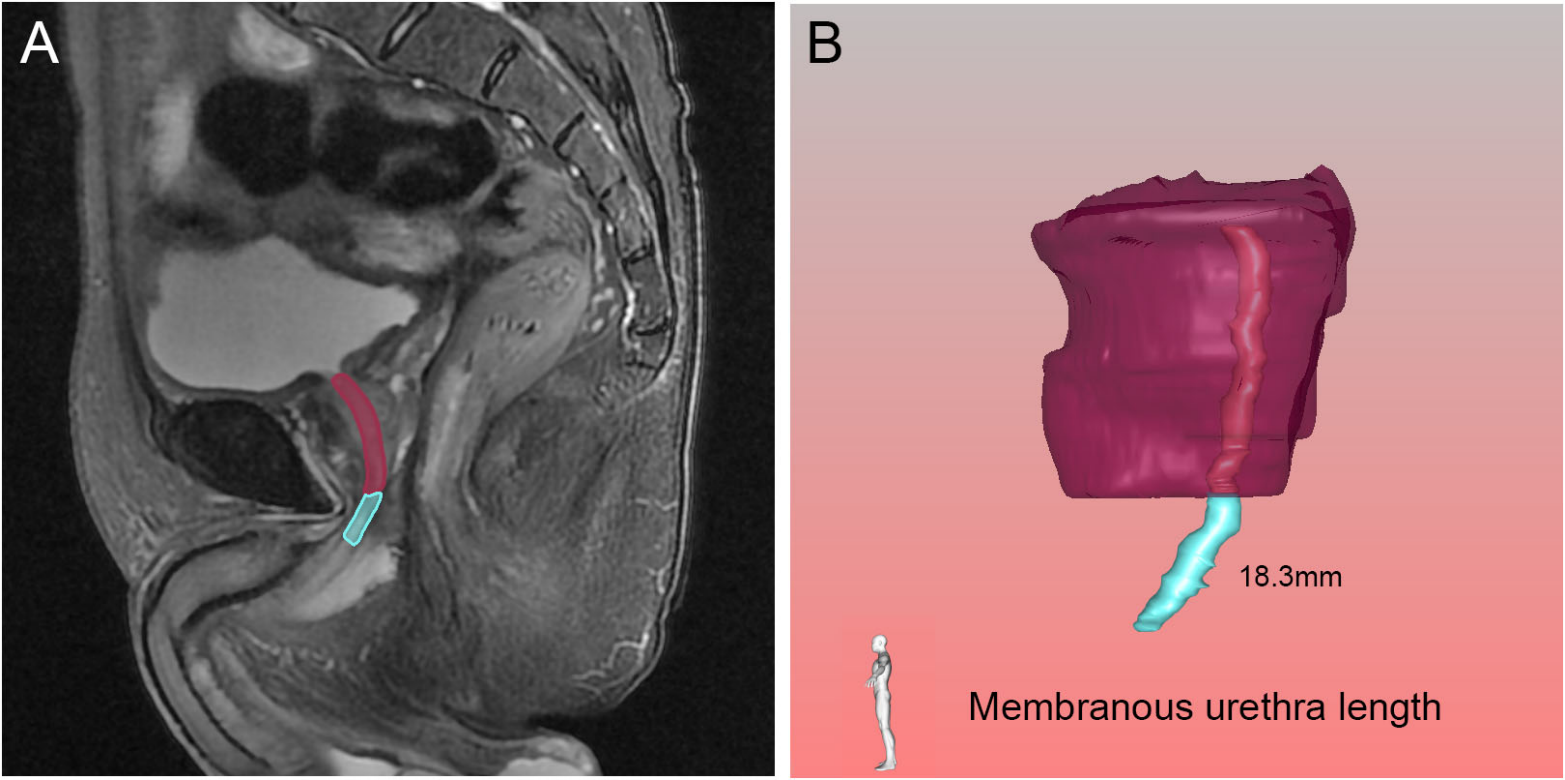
Membranous urethra length (MUL) in the sagittal (A) view on magnetic resonance imaging, and three-dimensional visualization technique (B). The pink, blue and purple structures represented the prostatic urethra, membranous urethra and prostate respectively.
